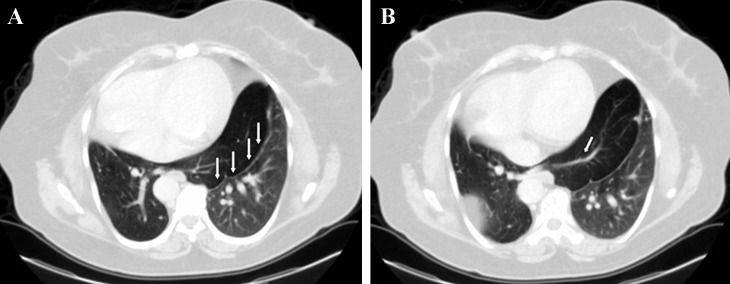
CT images in parenchyma window in the same patient A, Herniation of the right basal lobe and isthmus is visible. Herniated lobe is hyperinflated and surrounded by pleural membrane (arrows). B, Lobe bronchi contains fluid attenuation and extends to the herniated lobe (arrow). Herniated lobe is hypodense due to air remains.
